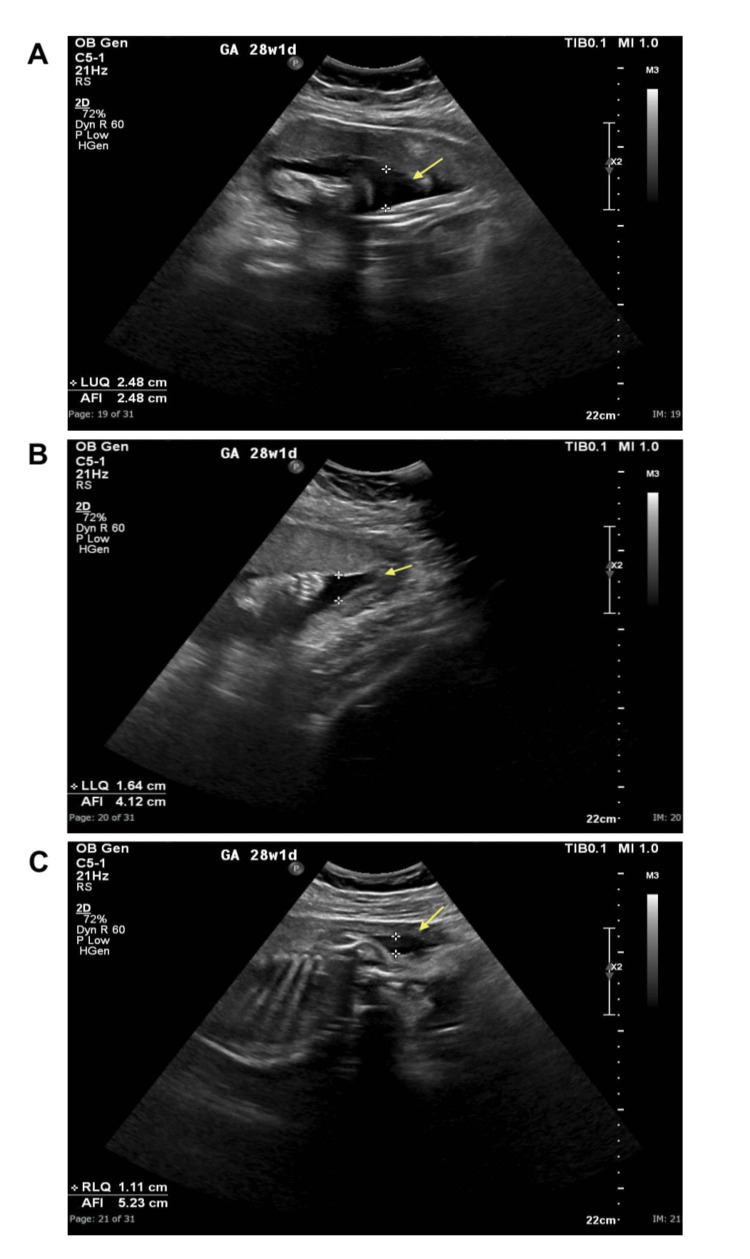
Obstetric ultrasound showing (A) the diameter of the LUQ fluid pocket measuring 2.48 cm, (B) the diameter of the LLQ fluid pocket measuring 1.64 cm, and (C) the diameter of the RLQ fluid pocket measuring 1.11 cm, with a total AFI of 5.2 cm, indicative of oligohydramnios. LUQ, left upper quadrant; LLQ, left lower quadrant; RLQ, right lower quadrant; AFI, amniotic fluid index
